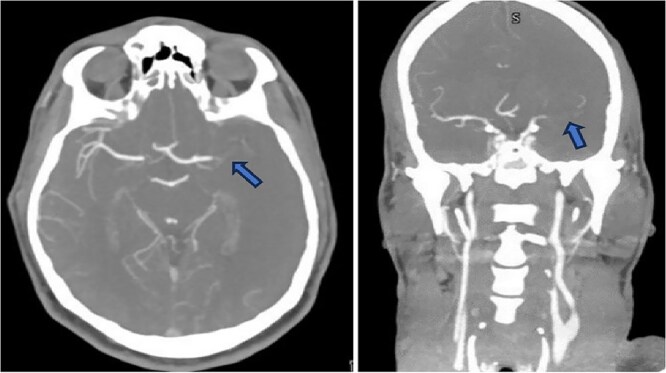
CT angiogram intracranial shows left MCA occlusion at the level of M1 segment. Rest of the anterior and posterior cerebral circulation appear opacified by contrast and is normal in caliber.
